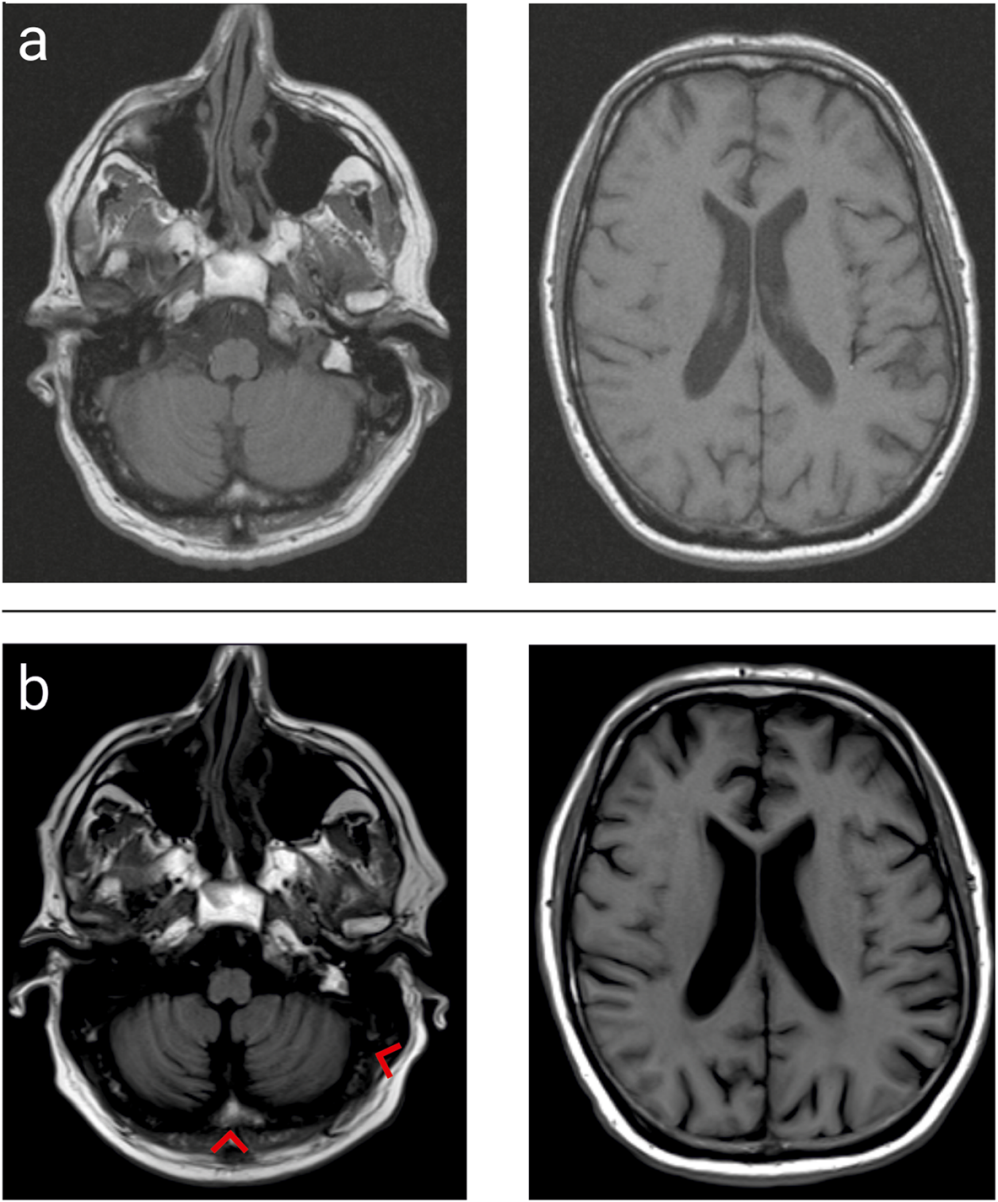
Magnet Resonance Imaging shows progressive atrophy of the cerebellum (indicated by the red arrows) on T1-weighted Turbo spin echo imaging at 10 months (a) and 16 months (b) after the onset of symptoms, indicative of progressive neurodegeneration. Figure designed with Corel Draw × 8 (Corel Corporation, Ottawa, Canada)
